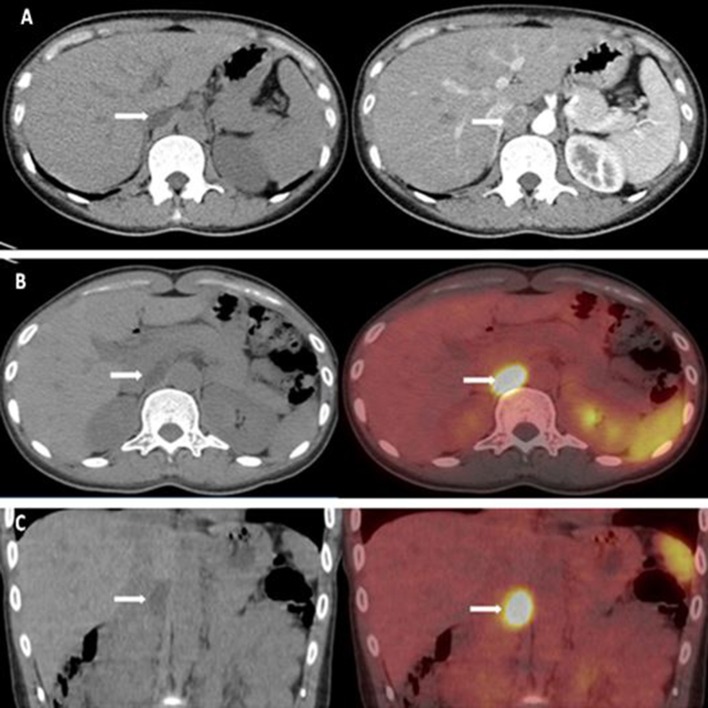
(A) CT scan revealed a 21 × 13 mm low-density mass (about 9 HU). The enhanced adrenal gland CT revealed a significant enhancement of the mass (about 70 HU). (B,C)
